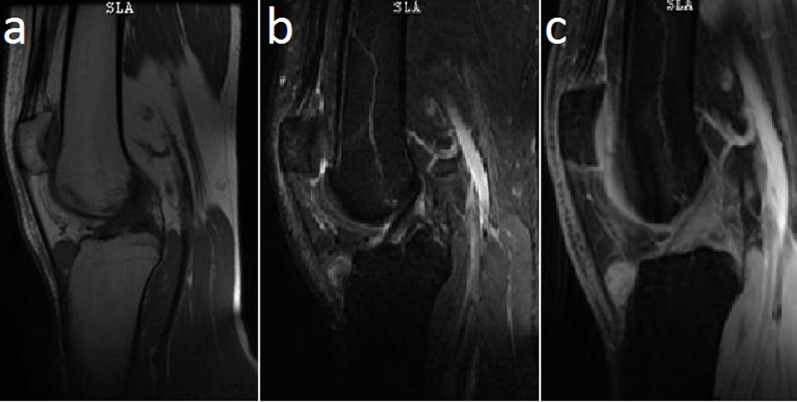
MRI showing a nodular encapsulated lesion localized in the infrapatellar Hoffa's fat pad. It presented as a hypo signal in T1 (a) and discrete T2 hyper signal interrupted by hyposignal punctuations (b) with intense homogeneous gadolinium enhancement (c)
